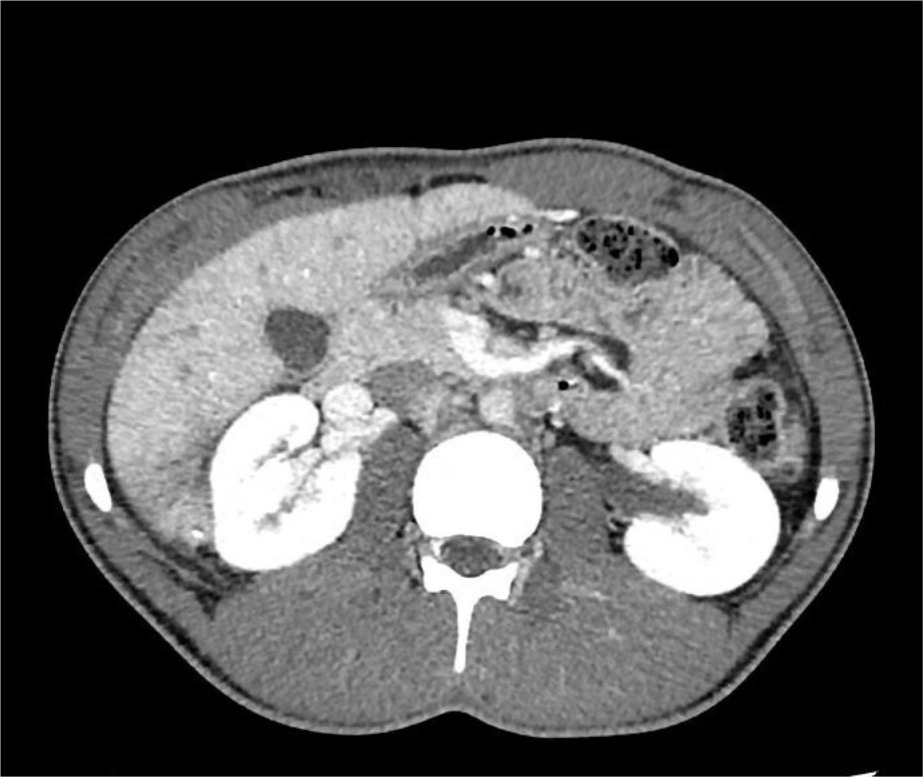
Abdominal MRI (after 4 months): Organized collection in S6 with wall thickening. The solid rim (high T2 signal intensity) demonstrates marked post-contrast enhancement.
